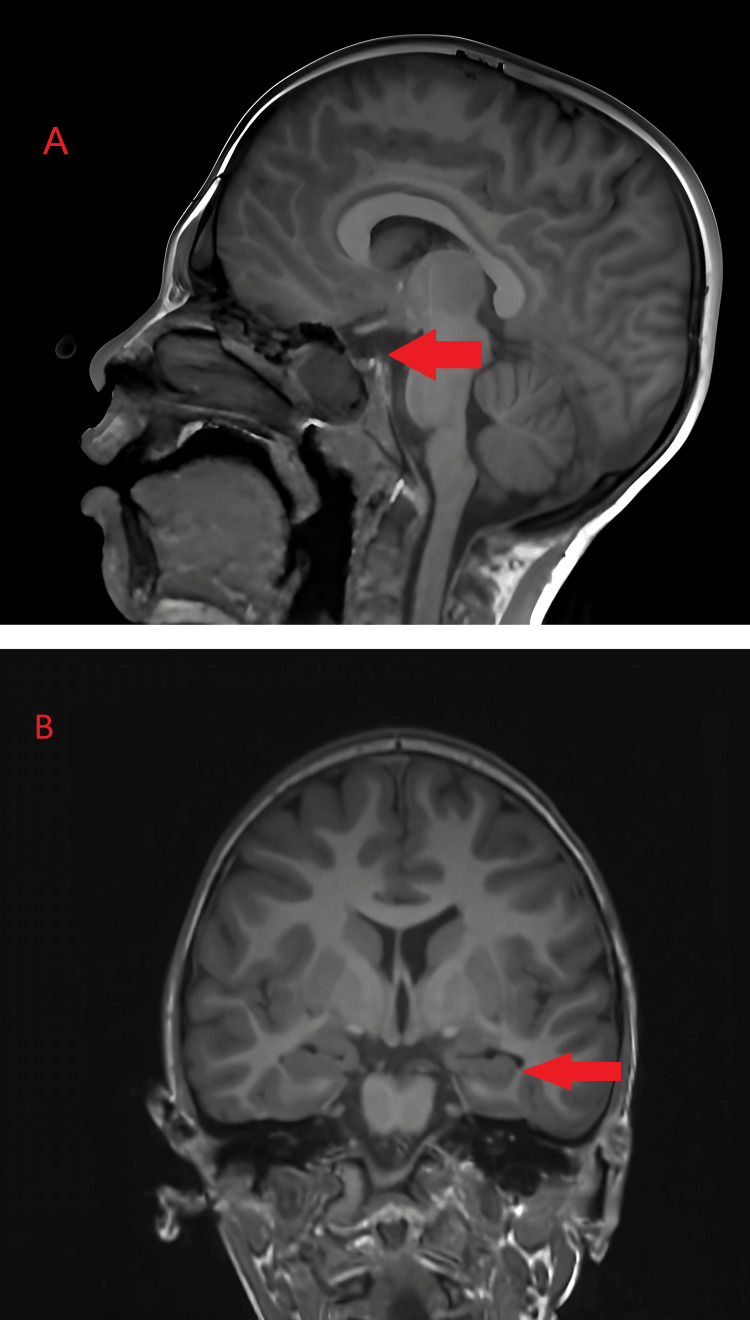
Magnetic resonance imaging (MRI) demonstrating structural abnormalities in the brain T2-weighted magnetic resonance imaging (MRI) showing (A) sagittal section showing a hypointense region in the posterior fossa (red arrow), suggestive of hypoplasia of the pituitary; (B) coronal section showing hippocampal gliosis marked by increased signal intensity in the hippocampal region (red arrow).
